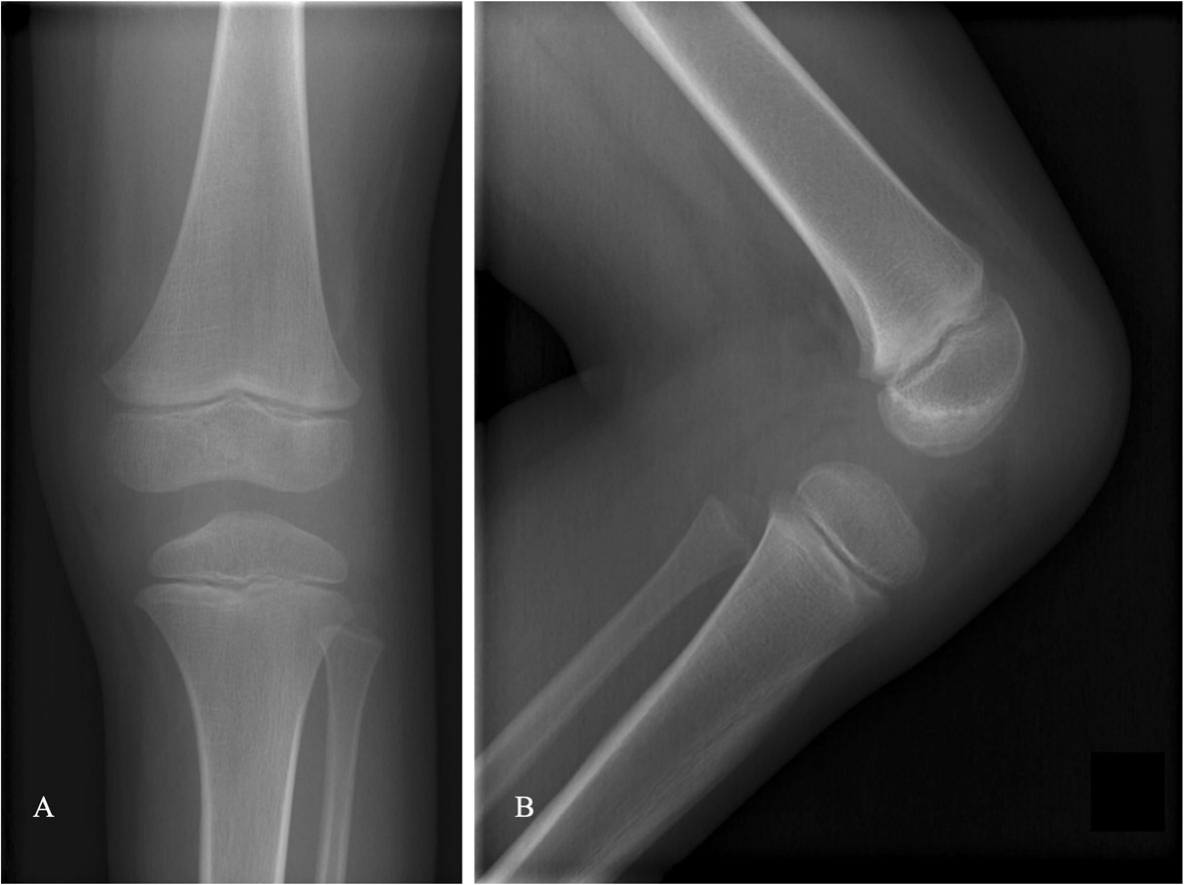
Plain radiograph of the concerned knee showing non-specific findings
